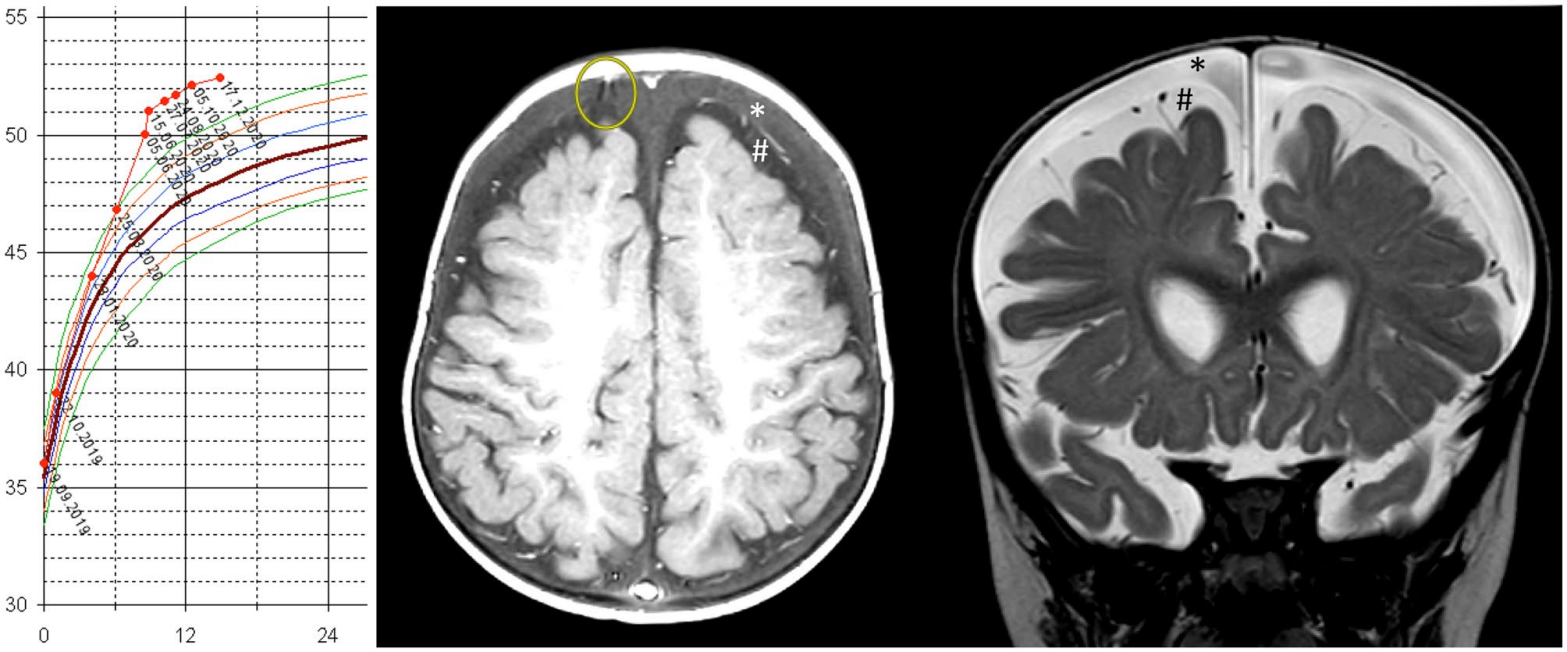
10-month-old boy presenting with a slowly rising head circumference visible at 6 months of age when reaching the 97th percentile (P97). At 8 months of age, his head was 2 cm above P97; the child was without symptoms. Within 10 days, his head circumference rose by 1 cm, and he showed signs and symptoms of raised intracranial pressure in with increasing severity (left picture). The MRIs (middle: axial T1 plus contrast and right: coronal T2) show an expanded subdural fluid space (*) above the expanded plus enlarged subarachnoid spaces (#) containing vessels and arachnoid membranes. The fact that the subarachnoid space is not compressed by the subdural fluid on top indicates that there is pressure equilibrium between both compartments. The yellow circle in the T1-weighted axial MRI depicts a bridging veins stretched by the expansion of the subdural space
